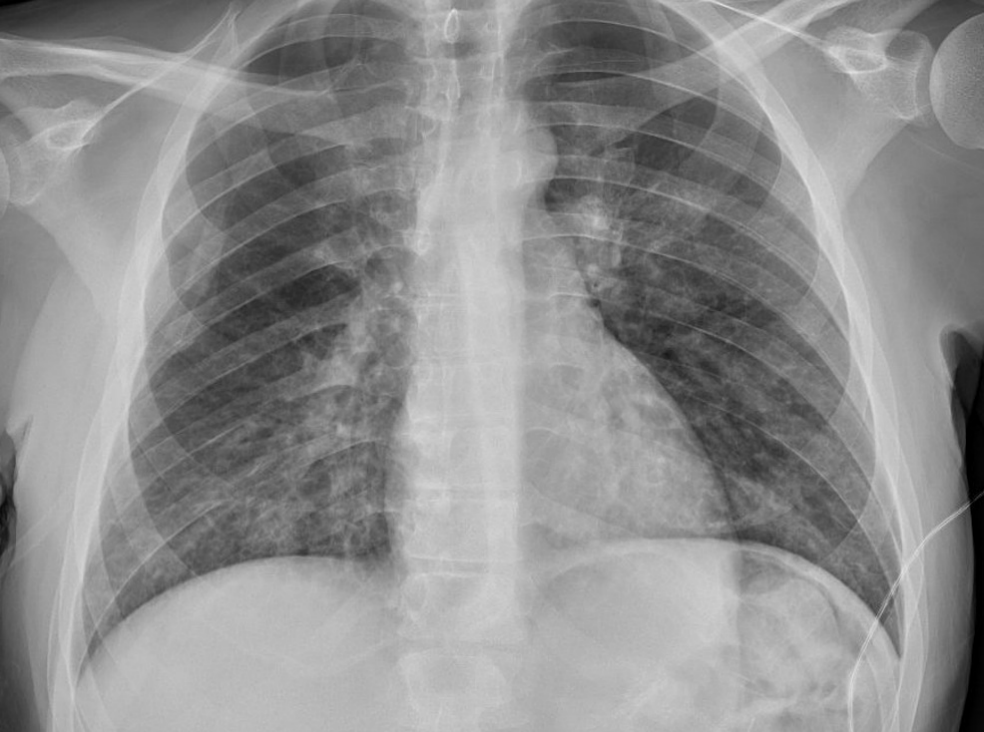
Chest radiograph after initial episode of desaturation, which did not show any abnormalities.
